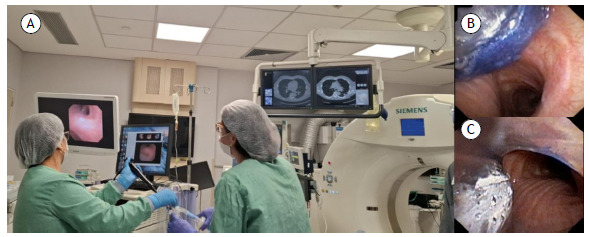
Placement of a double-lumen endotracheal tube for a CT-guided biopsy of a peripheral nodule with a high risk of bleeding. In A, patient undergoing intubation in the procedure room. In B and C, bronchoscopic view of the intubation procedure with a double-lumen endotracheal tube.
